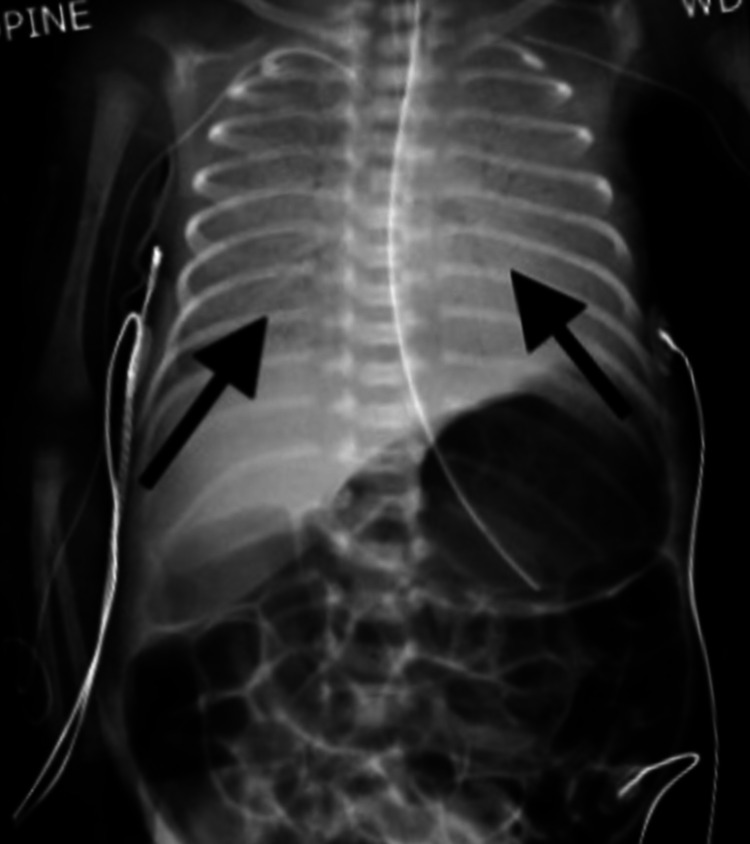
Chest/abdomen x-ray: AP view showing bilateral hazy opacities. No pleural effusion or pneumothorax was noted. AP: anteroposterior
